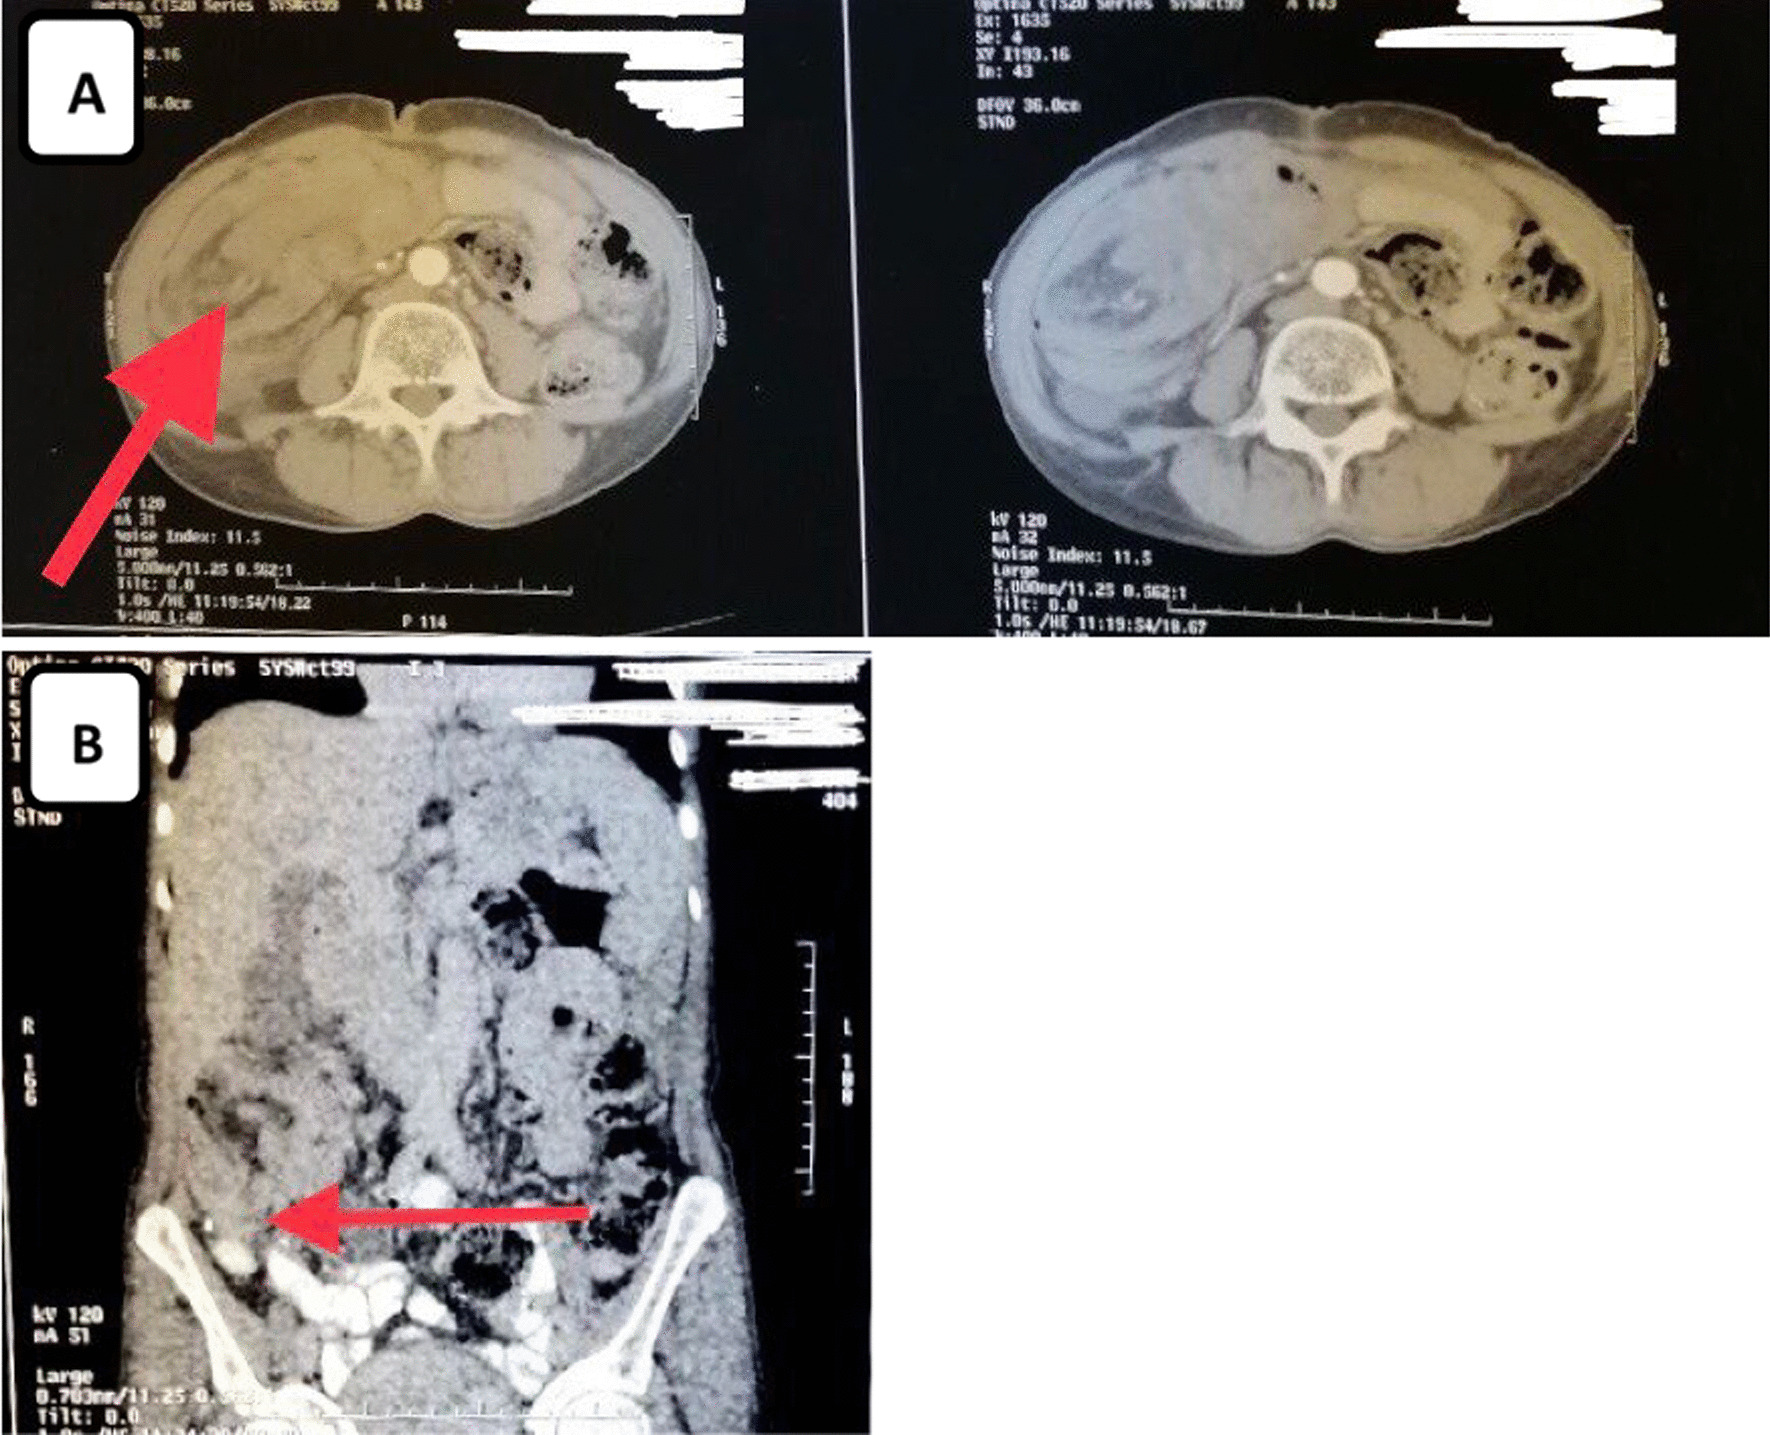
A Axial computed tomography of the abdomen shows the thickened wall of the intussuscipiens and the mass around the invaginating mesenteric fat (target sign) (the red arrow). B Coronal section of abdominal computed tomography shows the cecal mass (the red arrow) and thickened intestinal wall
